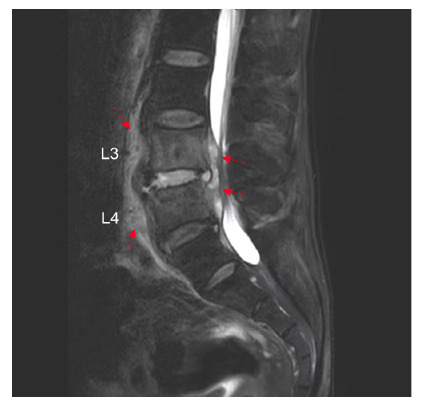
Magnetic resonance imaging (sagittal section) showing abnormal signals in the L3 and L4 vertebral bodies and the intervertebral disc accompanied by paravertebral and epidural abscesses (red arrows).
